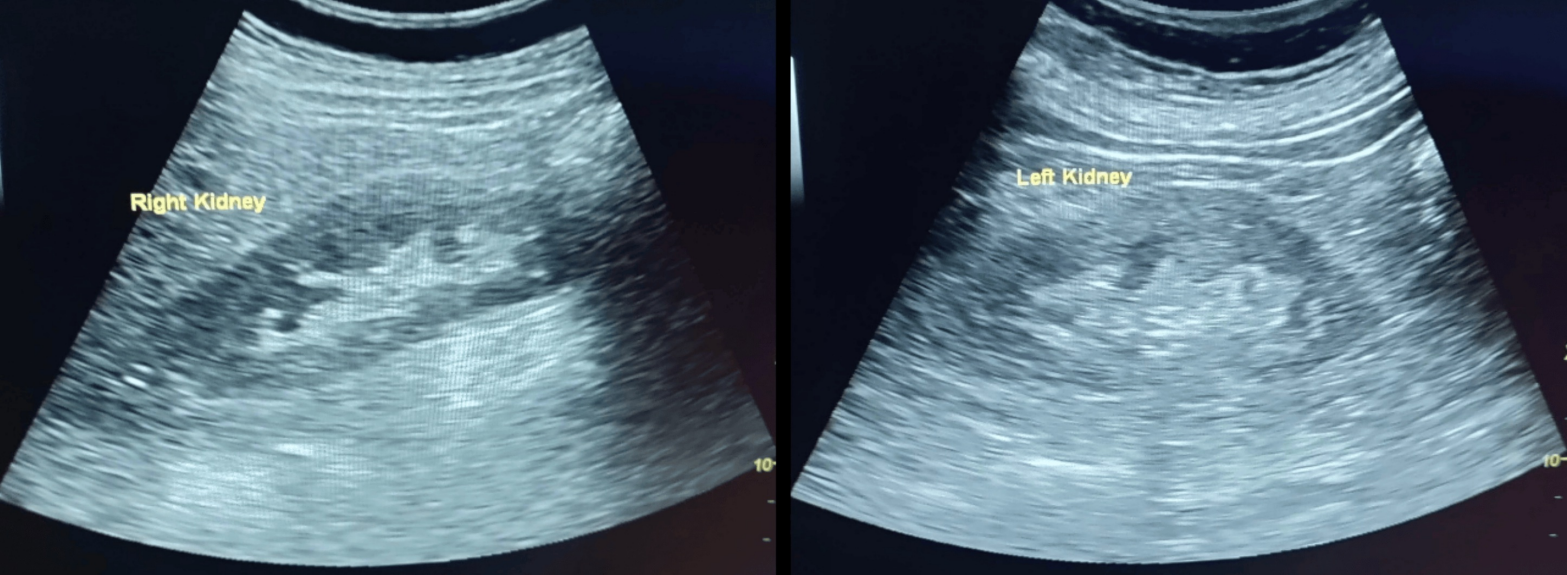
Ultrasound of the abdomen image showing both kidneys
